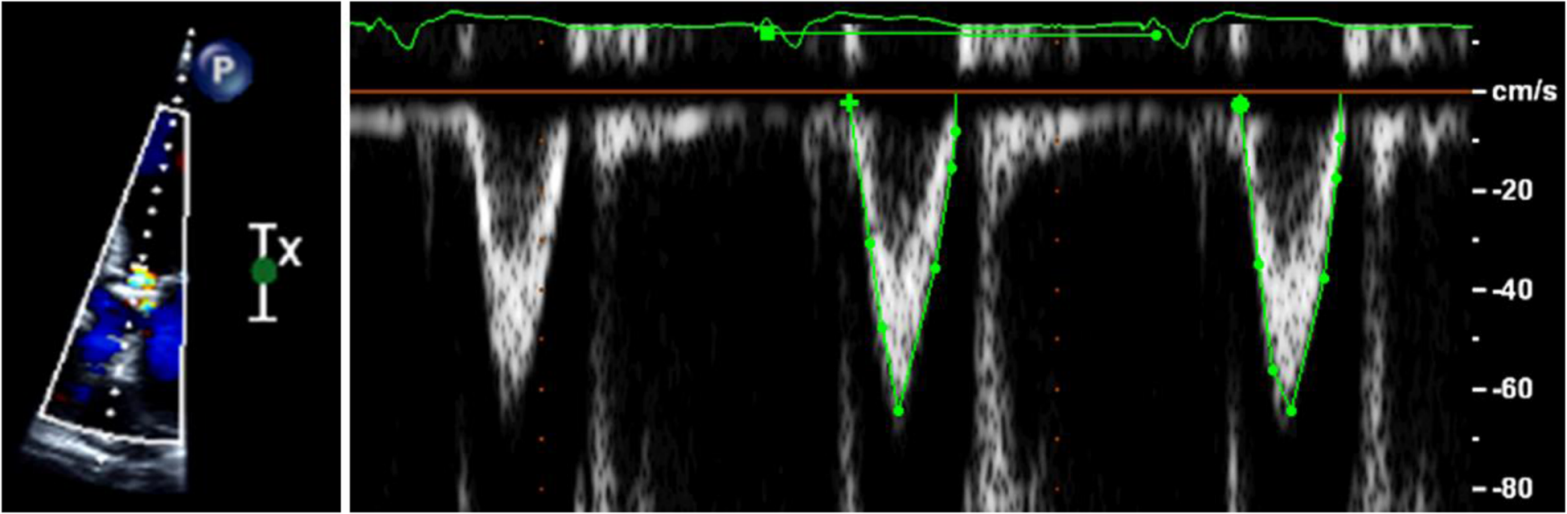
Left ventricular outflow tract stroke distance measured by pulsed wave Doppler echocardiography. Legend: Doppler signal from the apical view is directed parallel to flow through the left ventricular outflow tract and velocity time integral measured by hand planimetry
